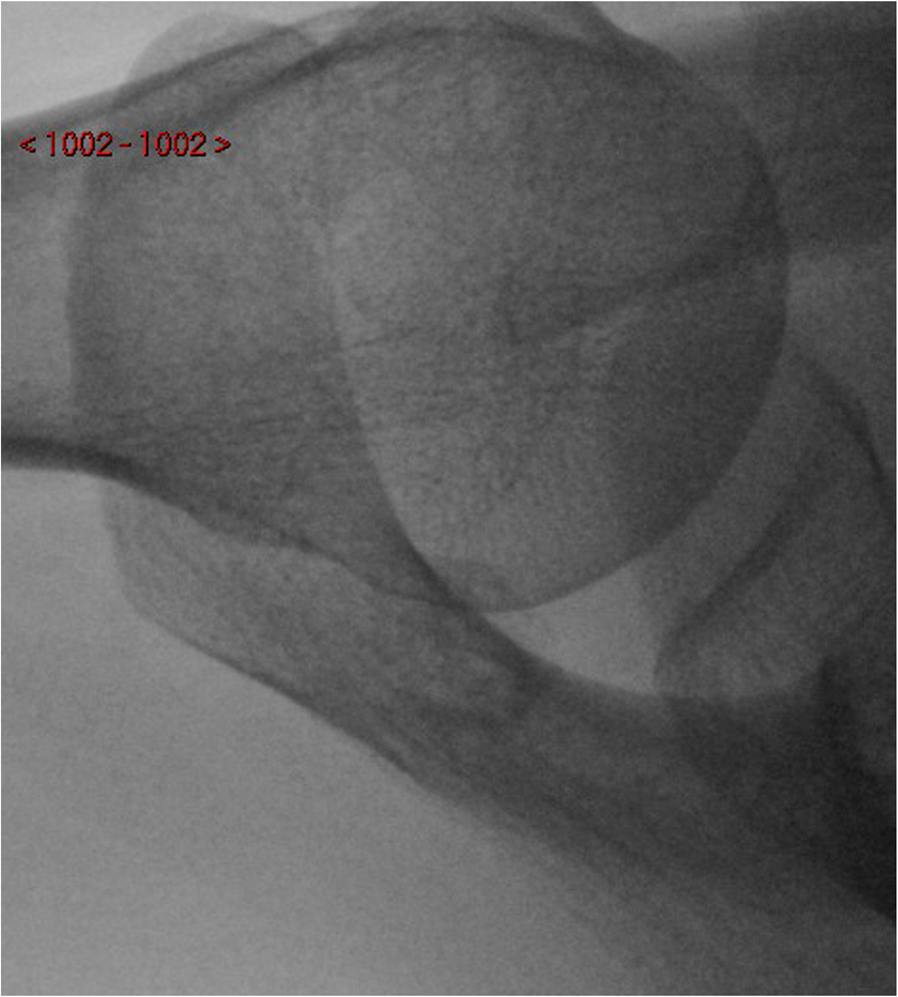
Axial radiograph of the left shoulder.
